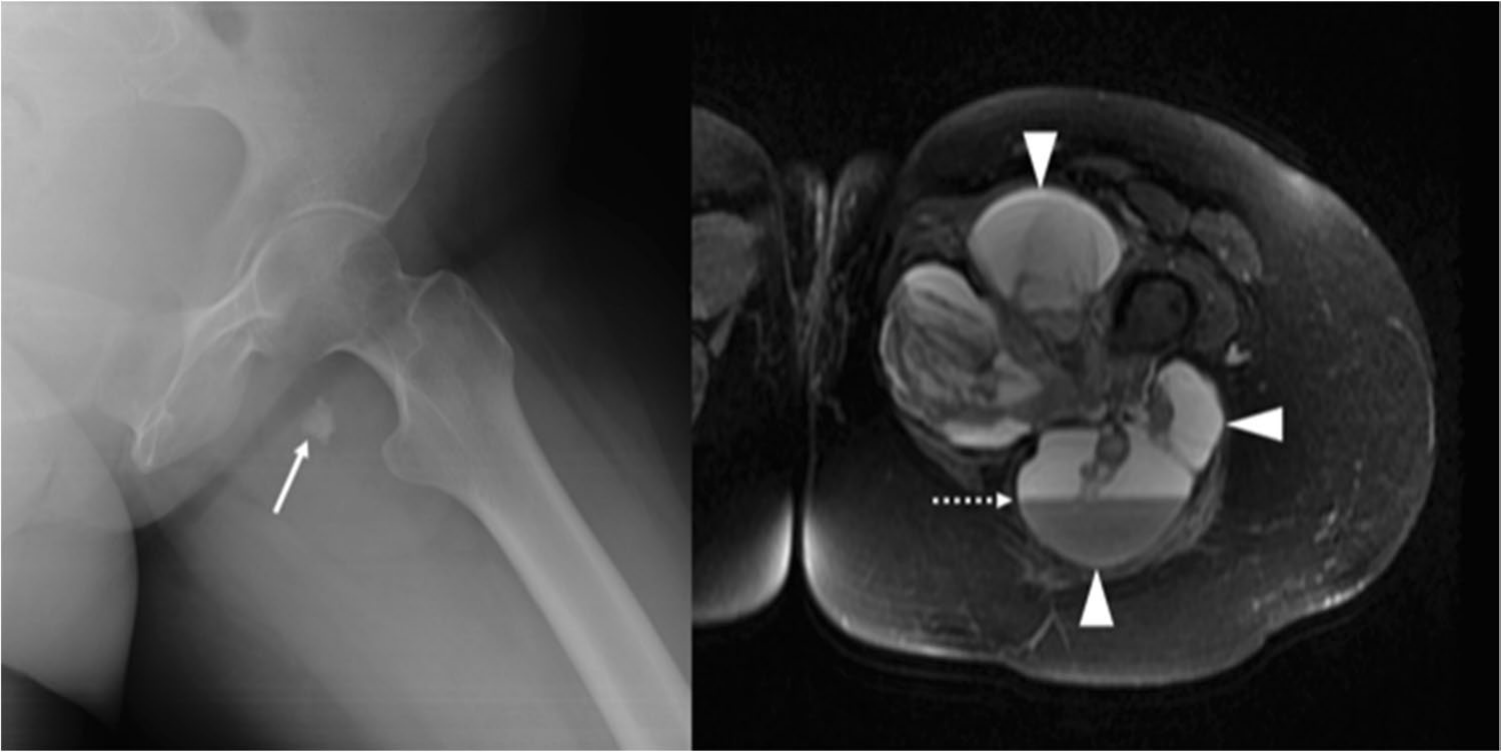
Conventional radiograph (left) and T2w axial fat-saturated MRI (right) of the left thigh in 32-year-old female with synovial sarcoma, showed calcific area (arrow), and the so-called “bowl of grapes” appearance with rounded cystic necrotic/hemorrhagic areas (arrowheads), containing a large fluid–fluid level (dotted arrow)
